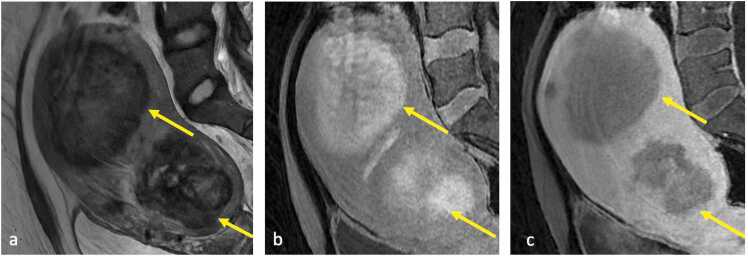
MRI findings of red degeneration of two uterine fibroids (yellow arrows). a) Sagittal T2 images demonstrates low signal in the fibroids b, c) Sagittal T1 fat saturated (FS) imaging pre and post contrast demonstrate high T1 signal in the fibroids that don’t enhance post gadolinium. DIAGNOSIS: Fibroid degeneration.
